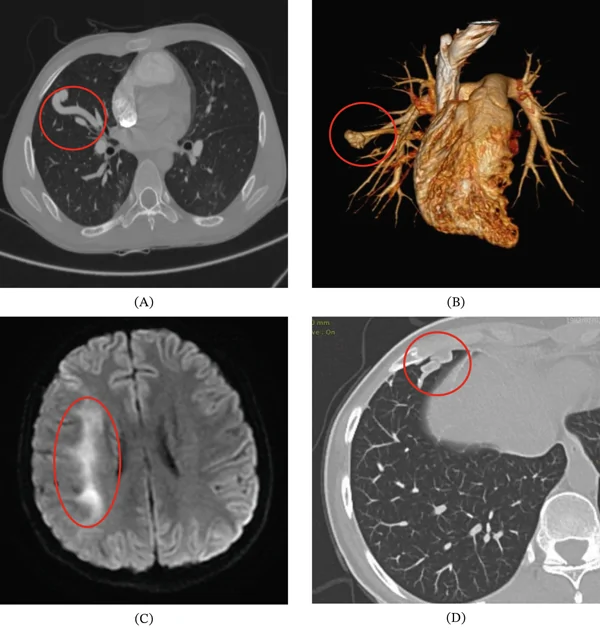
Imaging of Family 2. (A) Proband: Axial plane shows a large ductal shadow and nodular shadow in the right middle lobe of the lung. (B) Proband: VR shows an aneurysm sac, supplying pulmonary artery, and draining vein. (C) Proband: Cranial DWI shows high signal in the right corona radiata. (D) The proband′s mother: Axial plane shows tortuous and enlarged vascular shadow in the right middle lobe of the lung.
